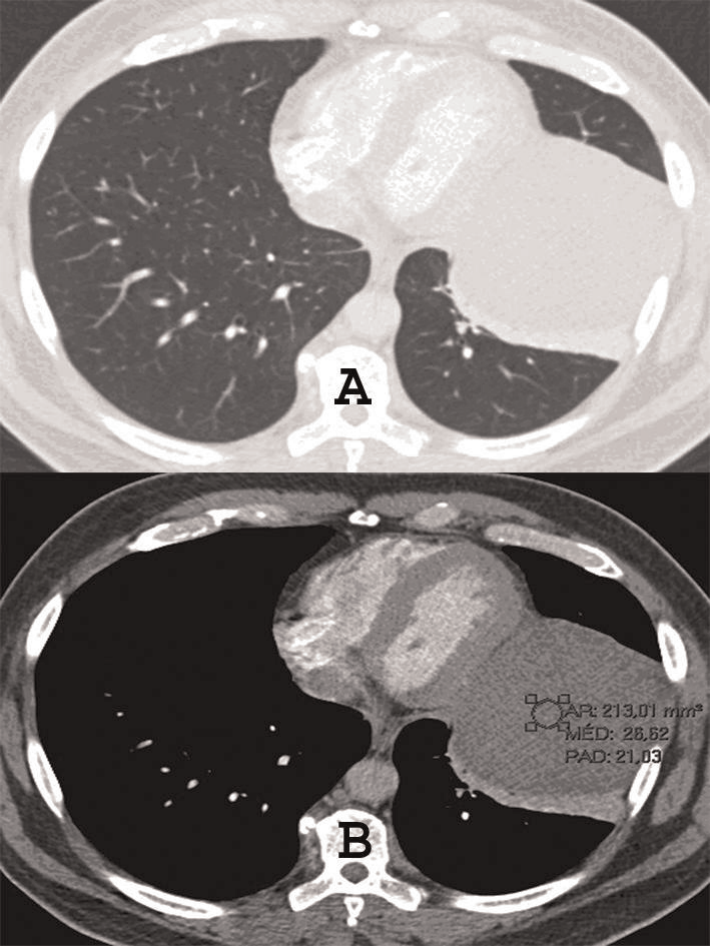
CT with (A) mediastinal and (B) lung windows shows predominantly a low attenuation mass (+27 UH) without enhancement in the left lower hemithorax.
